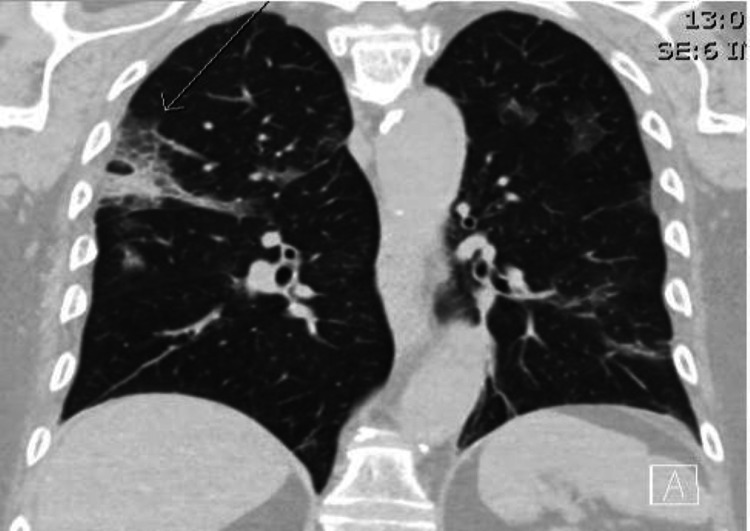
Spider web sign. CT coronal view showing ground-glass opacities, consolidation, and spider web sign (arrow). CT: computed tomography
